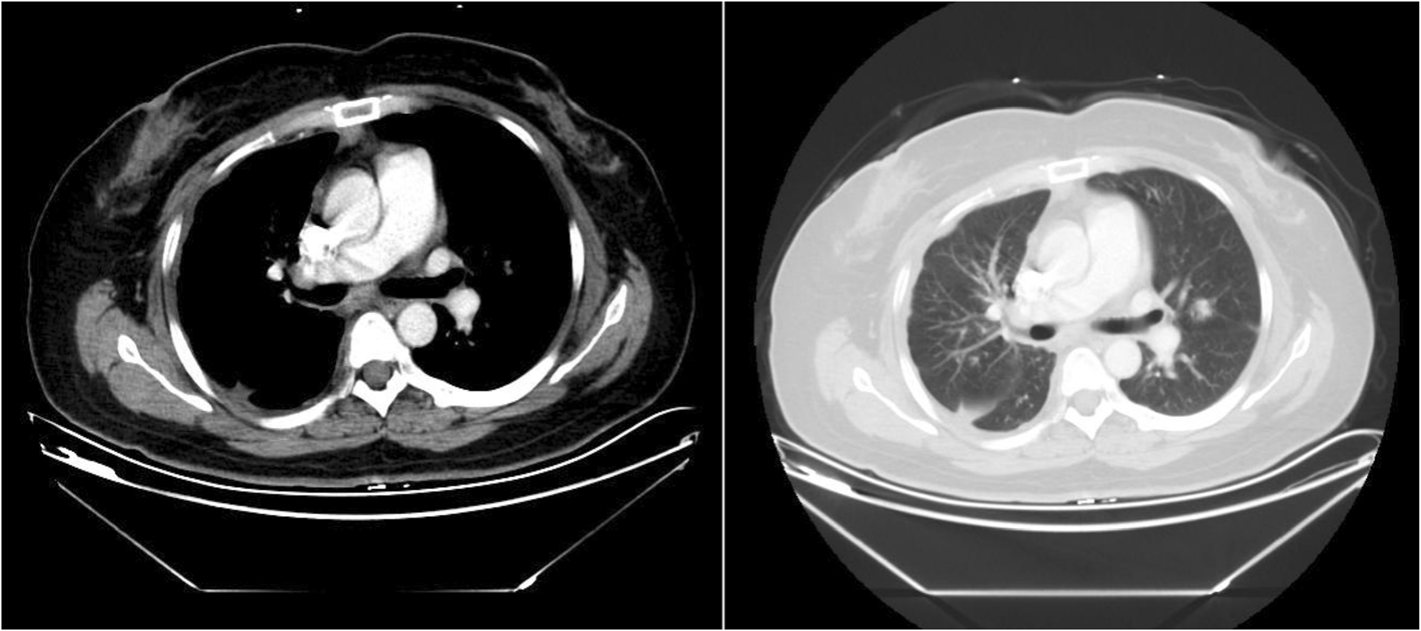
Enhanced chest computed tomography (CT) scan revealing bilateral pulmonary embolism, bilateral pulmonary infection, right pleural thickening and pleural effusion
